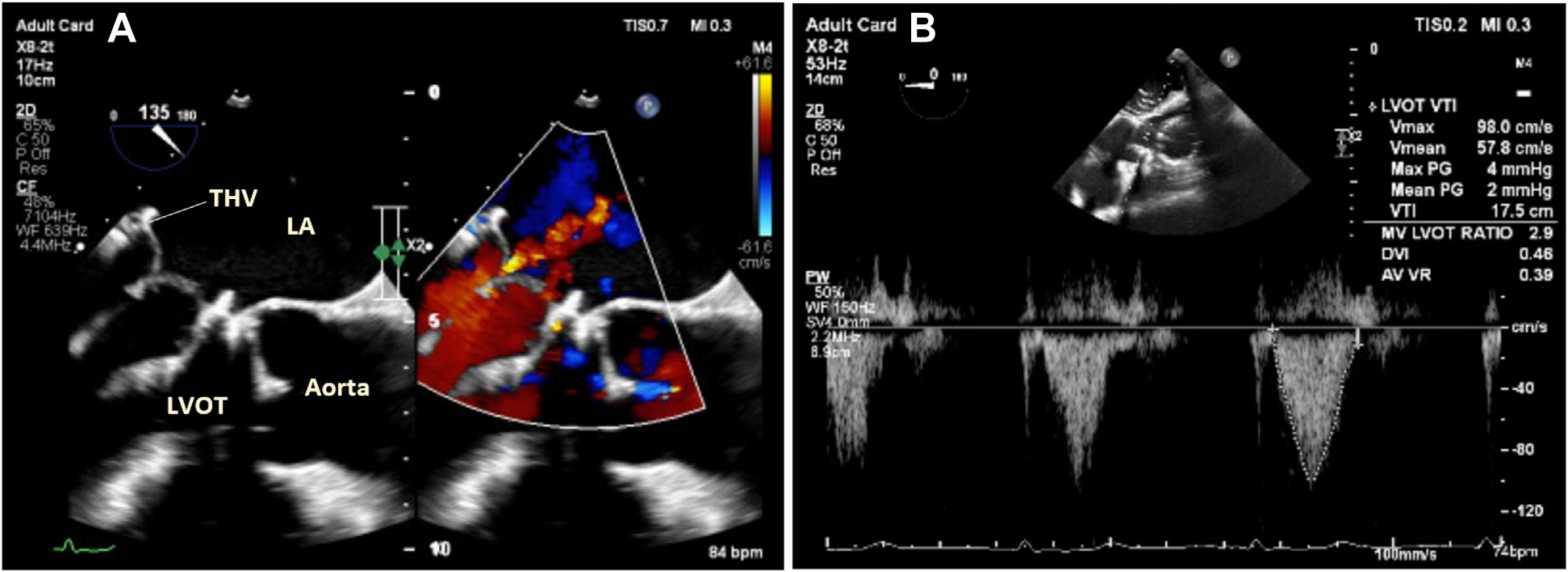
2-Dimensional Transesophageal Echocardiography The positioning of the transcatheter heart valve (THV) in relation to the left ventricular outflow tract (LVOT) with minimal transvalvular regurgitation (A) and a continuous-wave Doppler through the LVOT demonstrating no significant gradient (B). LA = left atrium.
